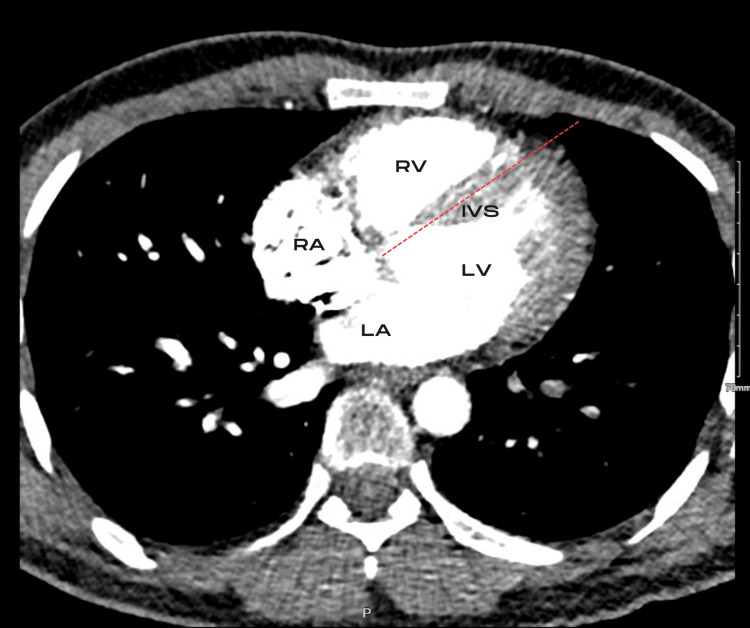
Evidence of right heart strain with flattening of the interventricular septum on CT pulmonary angiogram. RV: Right Ventricle; RA: Right Atrium; LV: Left Ventricle; LA: Left Atrium; IVS: Interventricular Septum.
